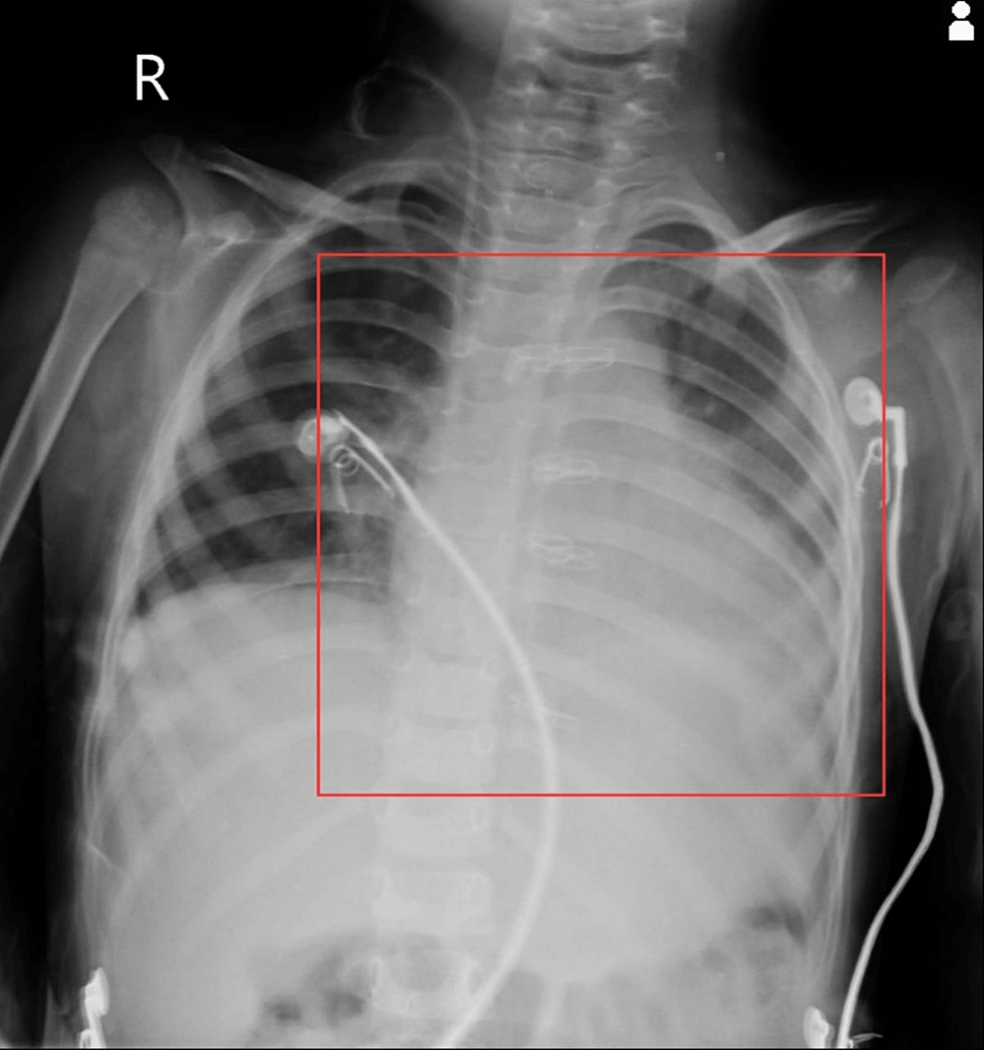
Postoperative chest radiograph Square represents chest leads and cardiomegaly
